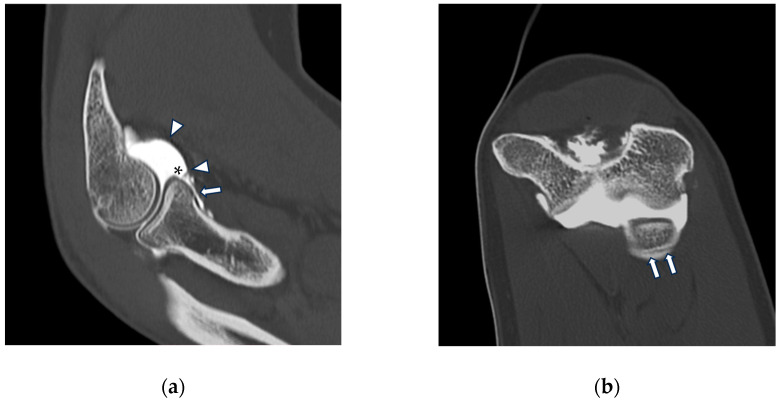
CT arthrography of a patient with overuse-related lateral elbow pain. (a) Sagittal image shows pathological widening of the radio-humeral and radio-annular recesses (white arrowheads) due to ligamentous laxity—in particular, the annular ligament (white arrow) is displaced distally to the proximal half of the radial head side, which also shows anterior cartilage thinning and synovial thickening (asterisk); (b) the corresponding coronal reformat may aid in precise localization of the proximal extremity of the annular ligament, which can be visualized as a hypodense band anterior to the radial head (white arrows); in this instance, it shows marked distal displacement.
